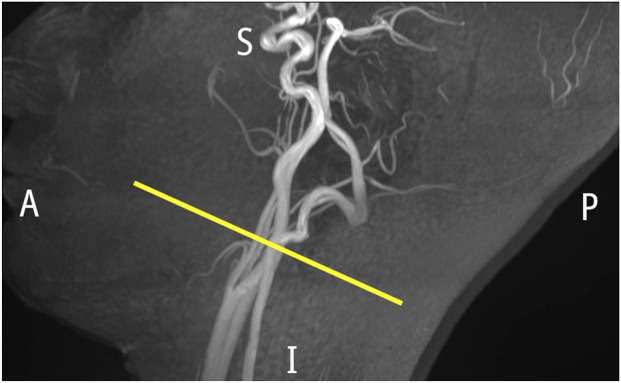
Maximum intensity projection of the arterial vessels (A, anterior; P, posterior; S, superior; I, inferior). Slice position for real-time MRI measurement is indicated by the yellow line.
